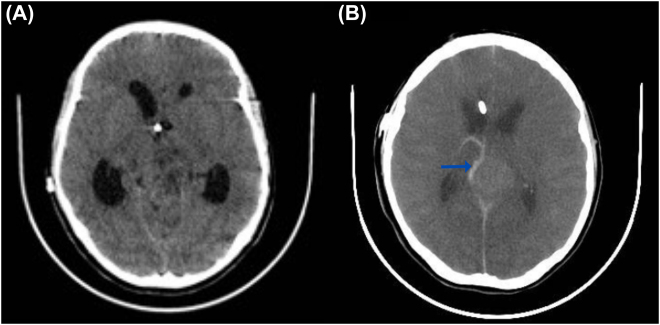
Brain computed tomography. (A) Loss of differentiation of the sulci and white matter with gray matter due to cerebral edema. (B) Loss of differentiation between the basal ganglia and the white matter; adjacent to mass, ganglioglioma, area of hemorrhage is observed (blue arrow).
